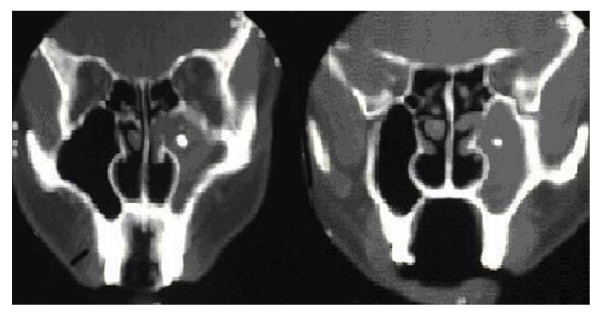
Computed tomography scan images showing complete opacification of left maxillary antrum along with widening of the ostium. A small, round high density body is also seen in the centre of the antrum.
